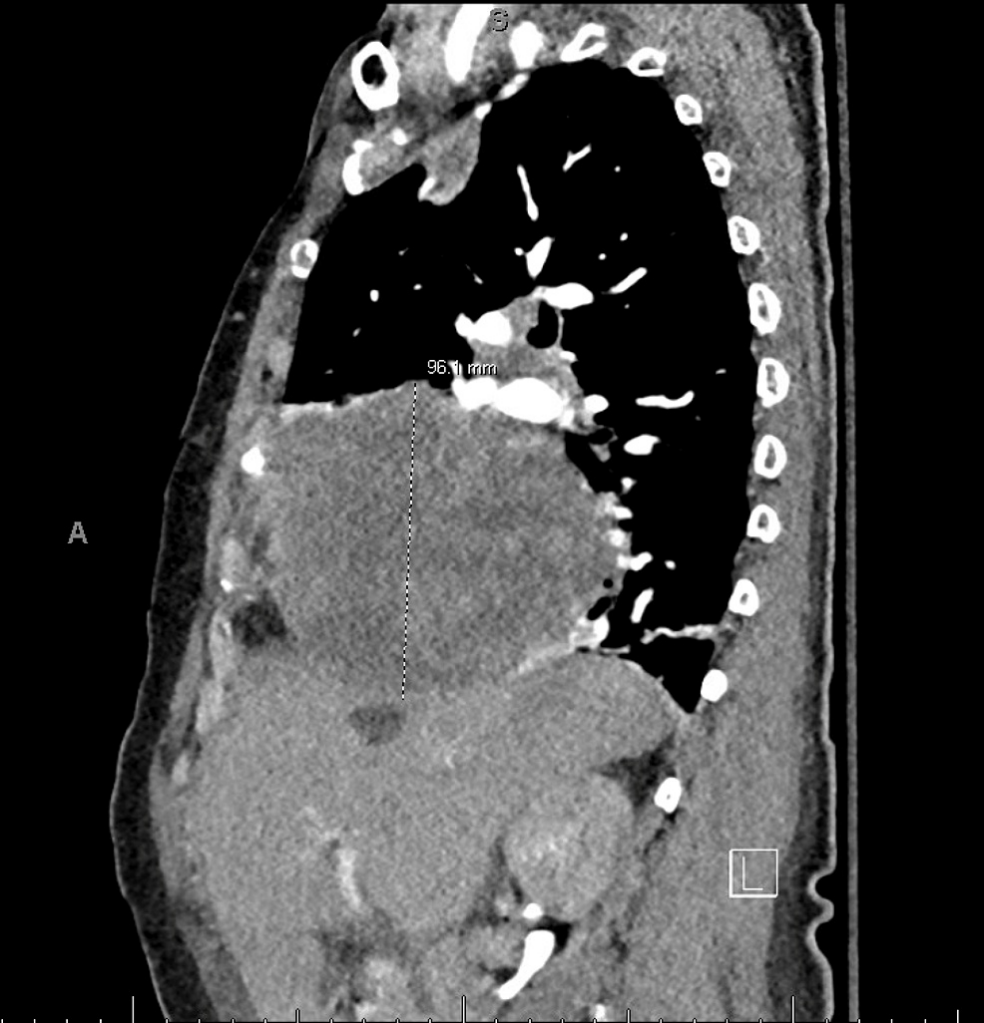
CT pulmonary abdomen pelvis (sagittal view). This figure shows a sagittal view of a CT pulmonary abdomen pelvis, showing a large mass occupying the anterior inferior half of the hemithorax that is approximately 12 cm traverse by 10 cm AP by 10 cm CC.
